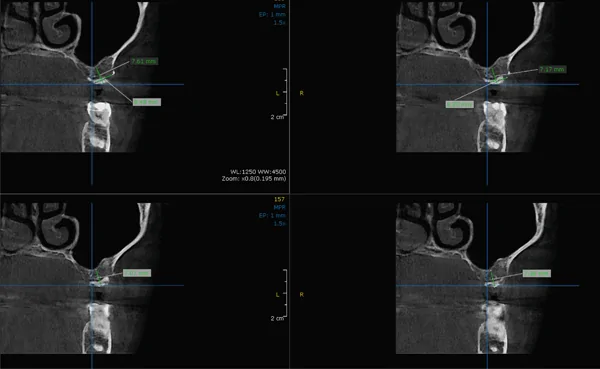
Assessment of alveolar bone support using cross‐sectional CBCT slices.
